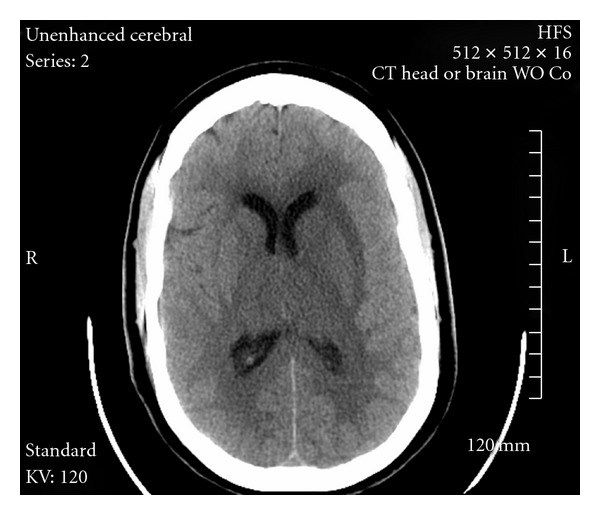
Noncontrast CT scan after 24 hours of intrasinus rt-PA.
